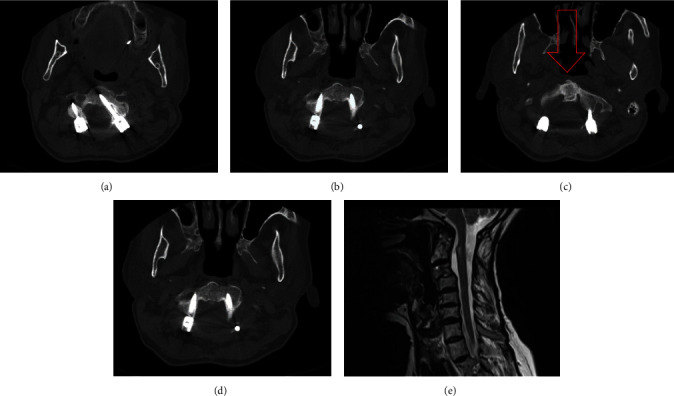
Cervical spinal CT-scan (a–d) and MRI (e) in 1-year follow-up, showing good fusion of odontoid process with stable position (arrow). CT, computed tomography; MRI, magnetic resonance imaging.
